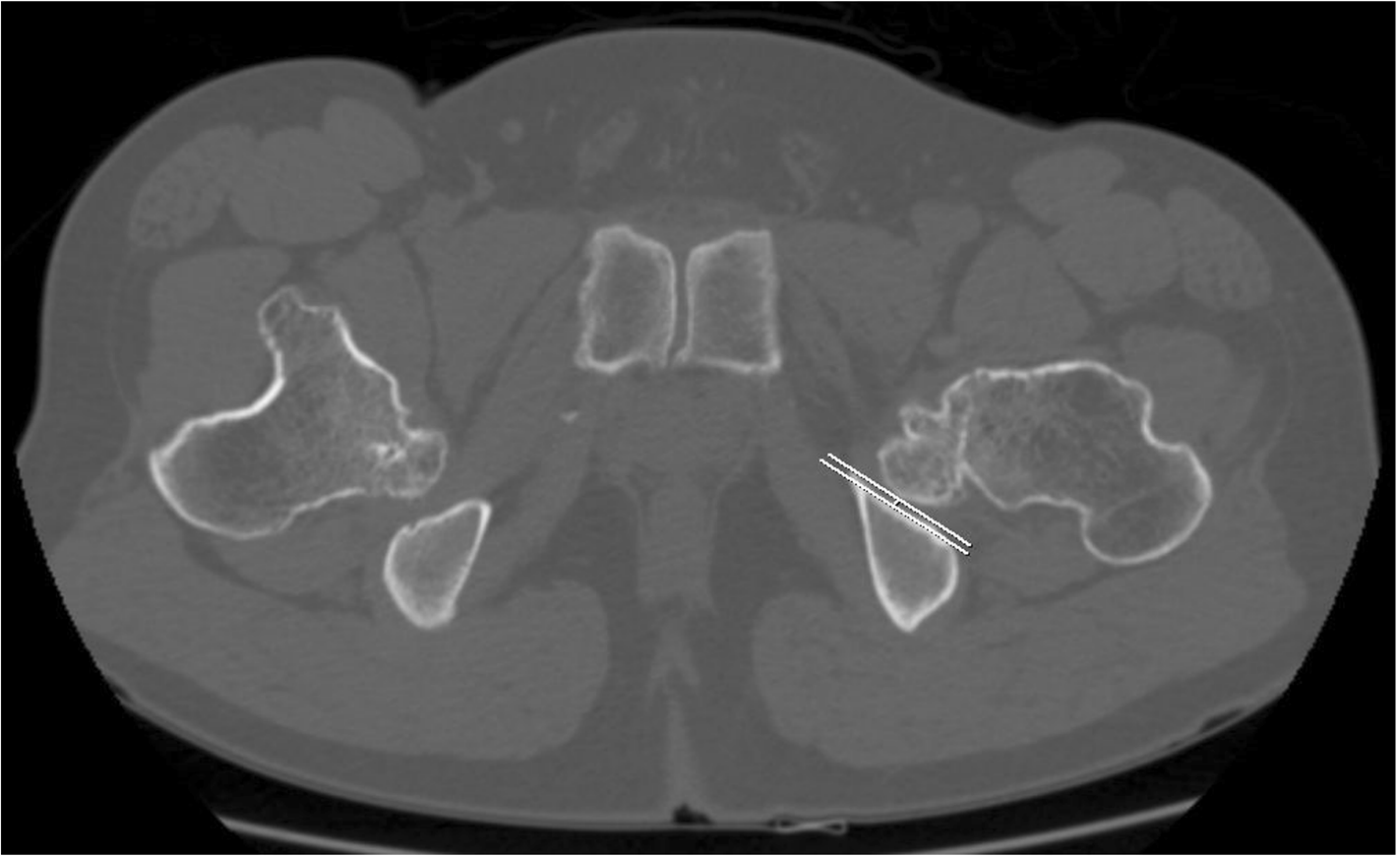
Measurement of minimum ischio-femoral distance to evaluate ischio-femoral impingement in a computed-tomographic study. The minimum ischio-femoral distance is determined by the nearest distance between the exostoses and ischium around the lesser trochanter area were measured at axial CT plane
